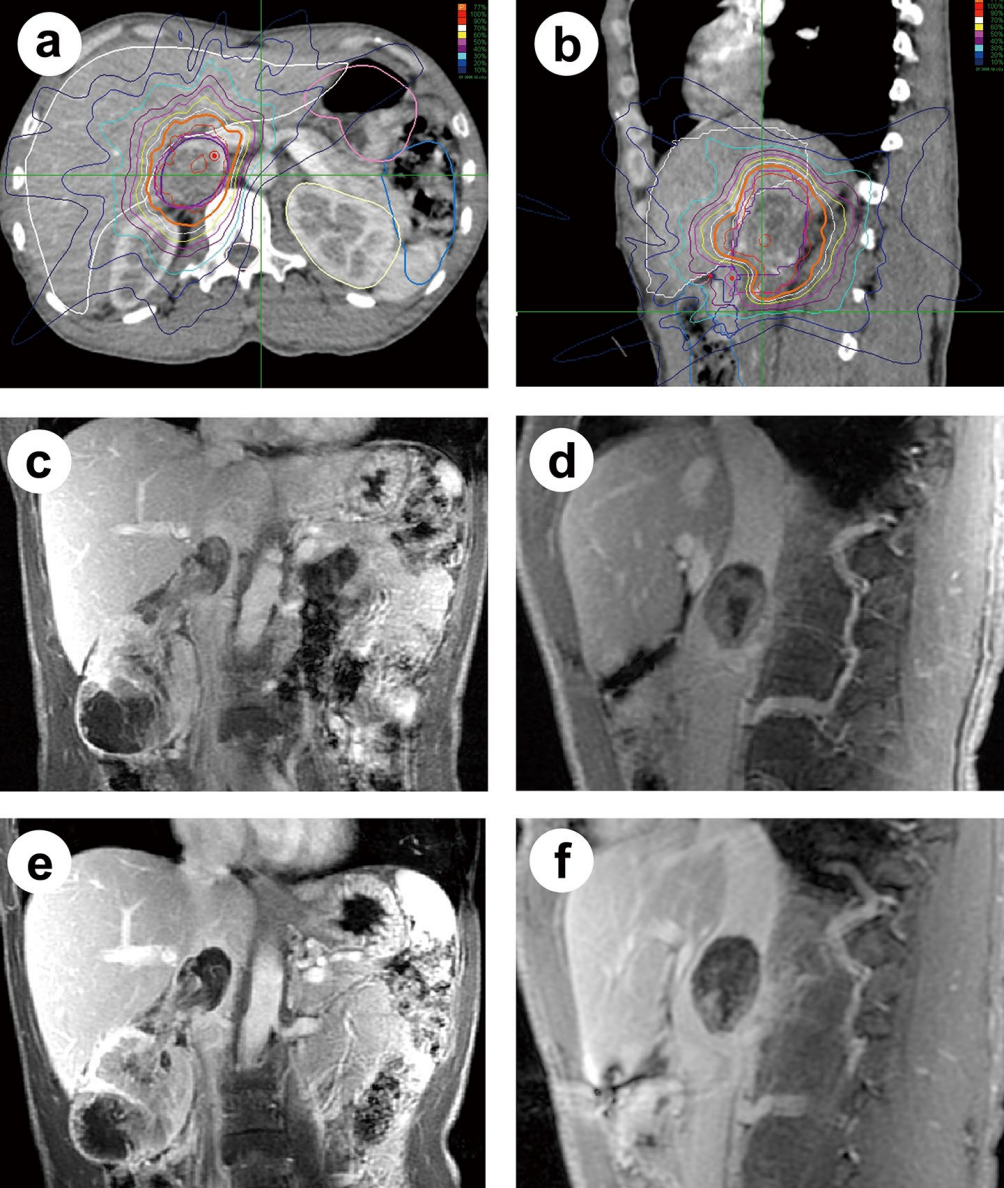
CT images showing the SABR treatment plan (a, b) and comparisons of IVC-TTs before (c, d) and after SABR (e, f). (a, b) The PTV of SABR on the cross-section and coronal planes. (c, d) Coronal views of the primary RCC tumor and Mayo grade II IVC-TT from a single patient before SABR. (e, f) CT images of the same primary tumor and IVC-TT after SABR, compared to those in c and d, respectively
